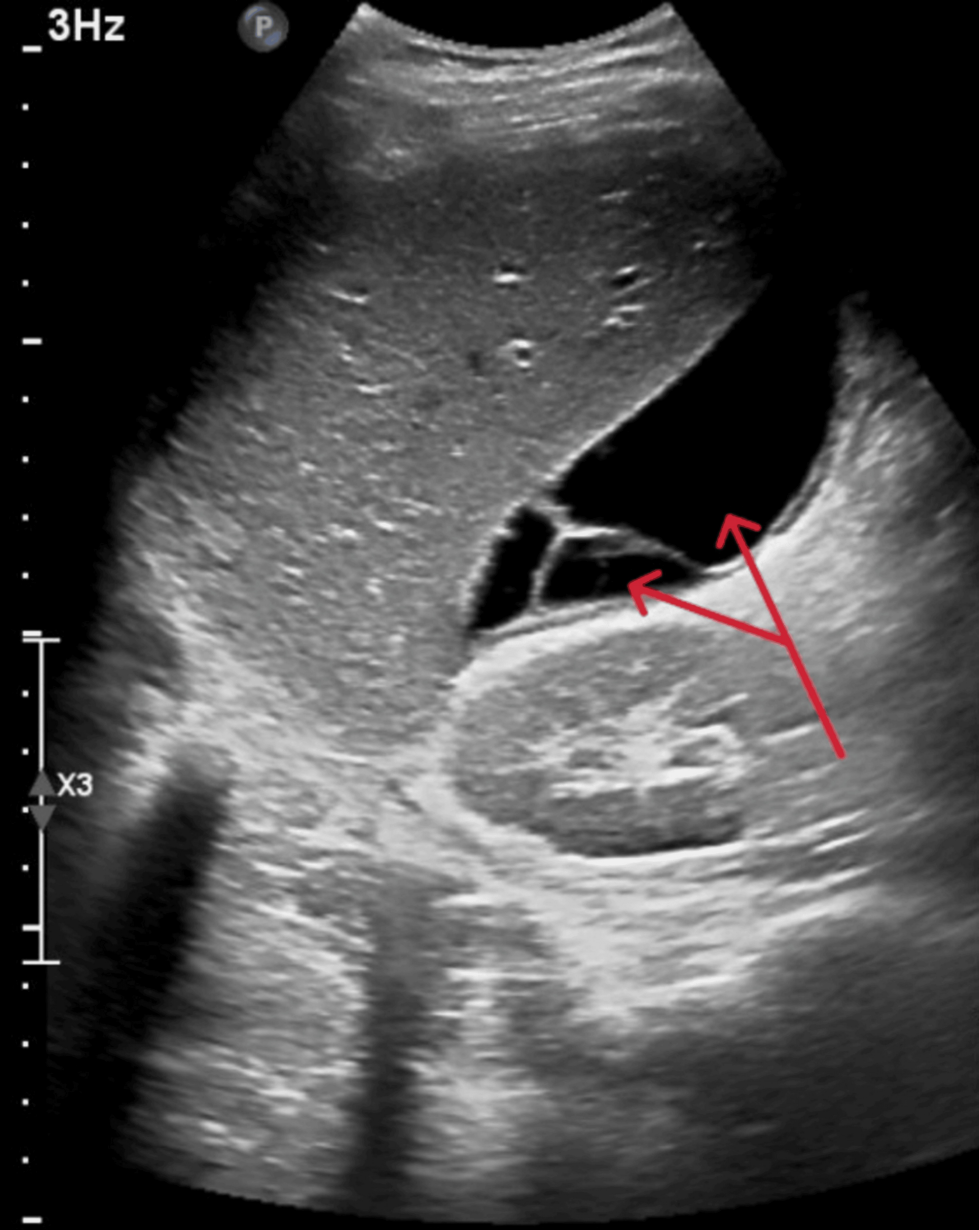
Ultrasound of the abdomen revealed subhepatic collection with septation (red arrows)
